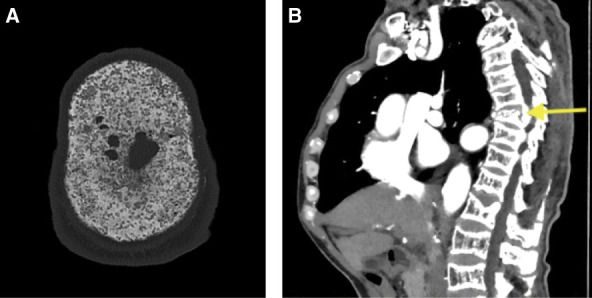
CT demonstrates changes consistent with severe tertiary hyperparathyroidism. (A) CT scan imaging demonstrates a mottled appearance of skull calvaria consistent with salt and pepper skull. (B) CT scan demonstrates rugger jersey spine and compression fracture. CT, computed tomography.
